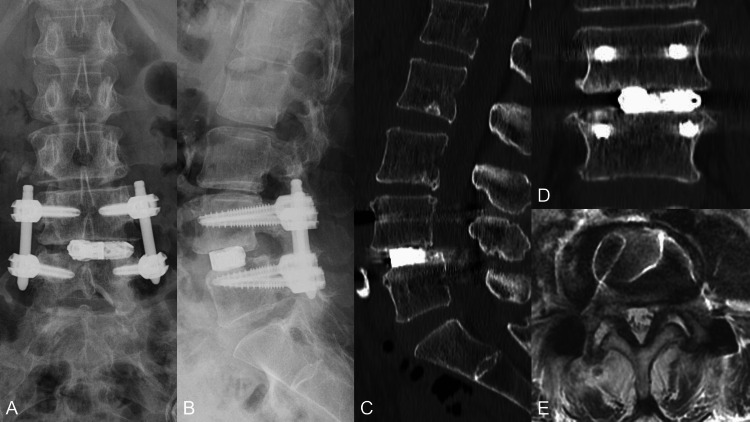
Postoperative imaging (A, B) Plain X-ray showed L4 spondylolisthesis was reduced; (C, D) Sagittal and axial CT scans showed that the cage was in sufficient contact with the L4/5 vertebral endplate; (E) Axial MRI at the L4/5 level showed the spinal canal area was expanded.
